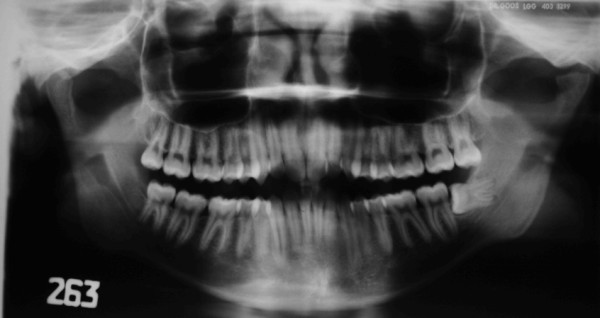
Pre-treatment panoramic radiograph showing normal morphology of right condyle and left condylar resorption.
